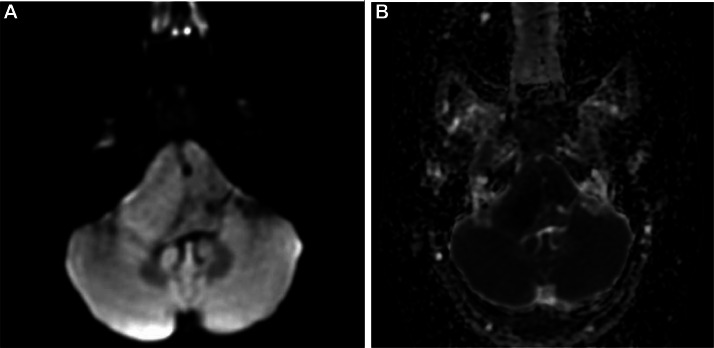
(A) DWI and (B) ADC map, show No diffusion restriction within the contents of lesion.
